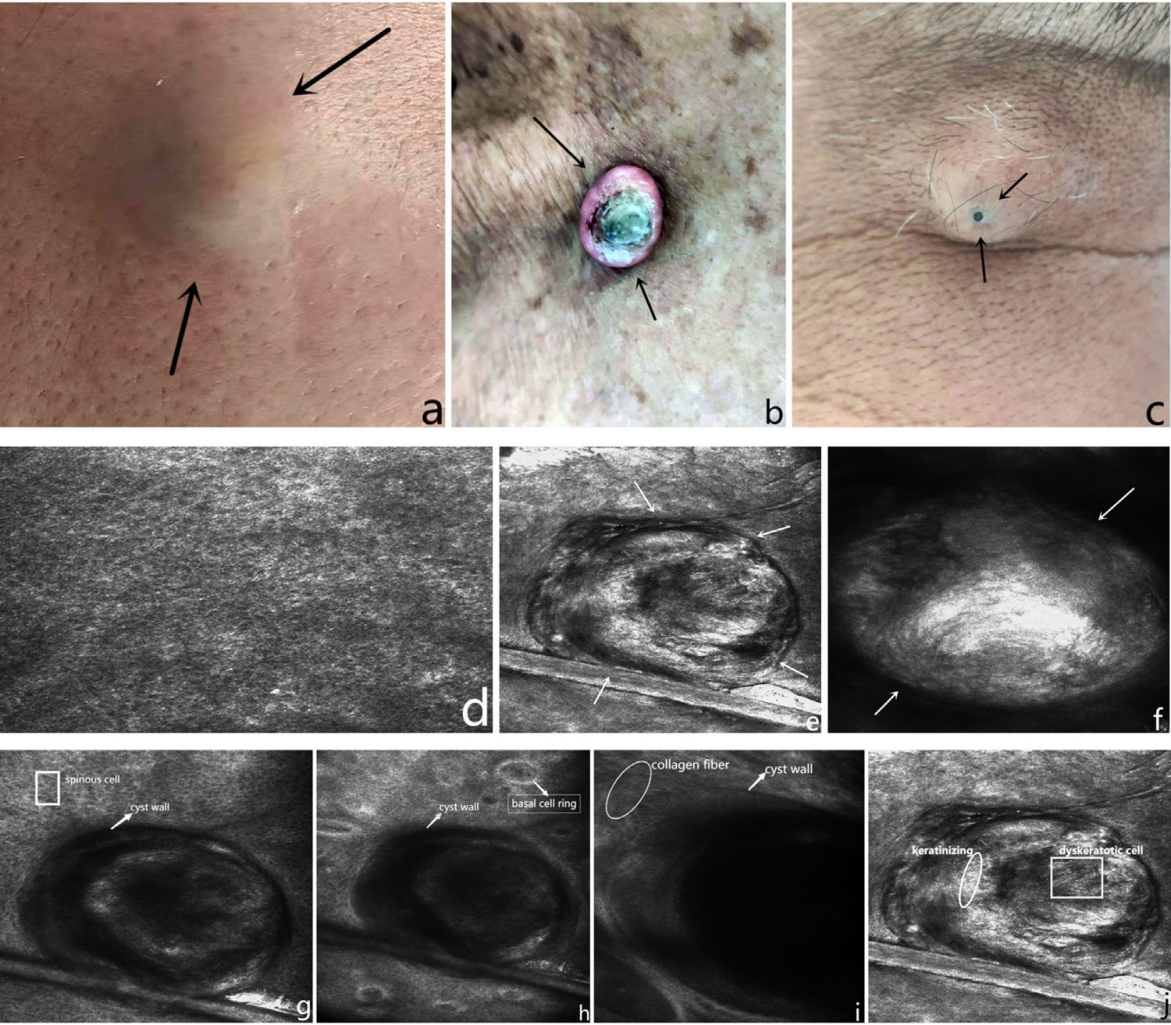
Clinical features and skin computed tomography (CT) image features of epidermoid cyst
